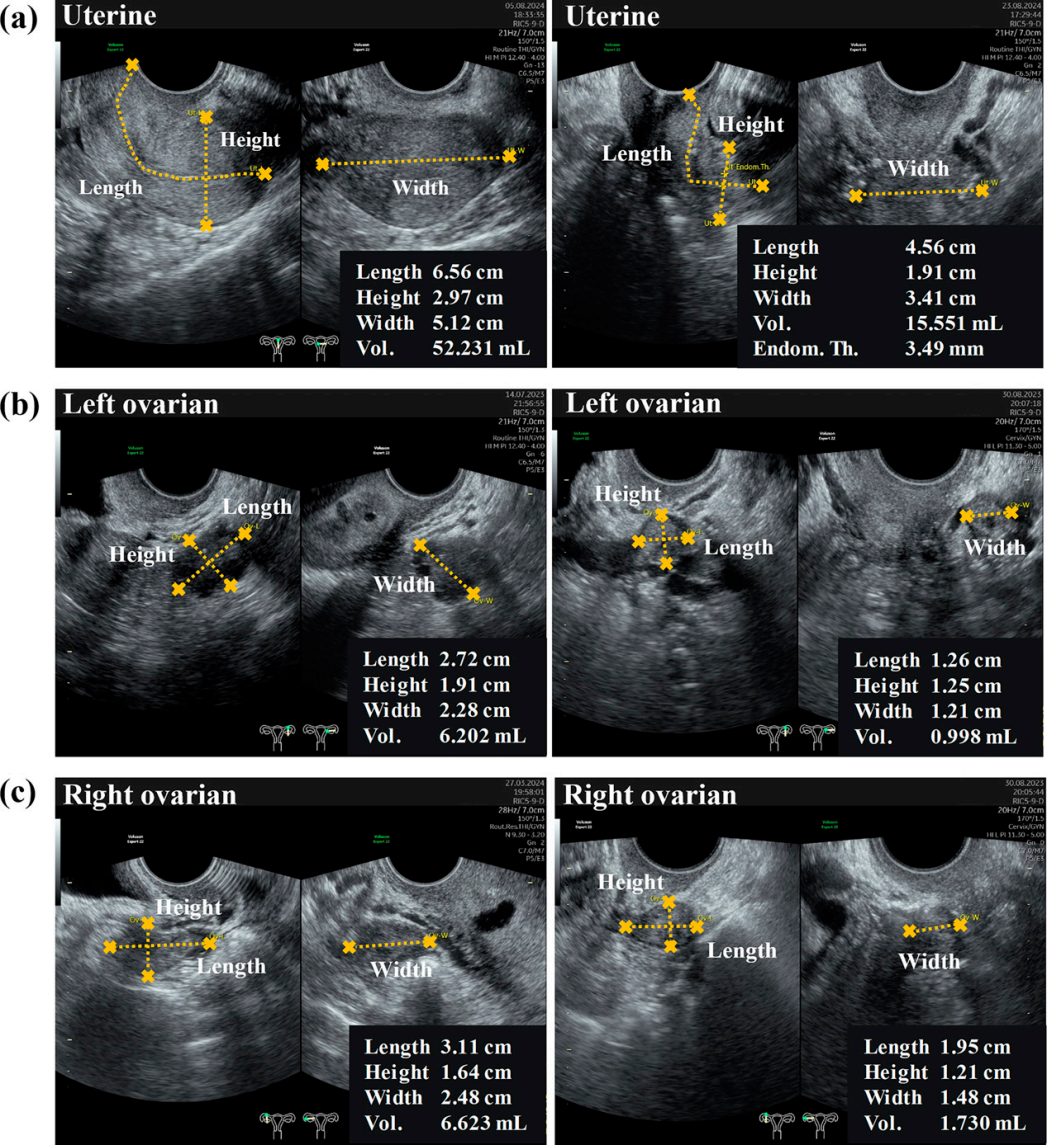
Ultrasonographic images of (a) uterus, (b) left ovary, and (c) right ovary. The left column shows organs in reproductive-age women (31–40 years old), while the right column depicts organs in postmenopausal women (>60 years old).
